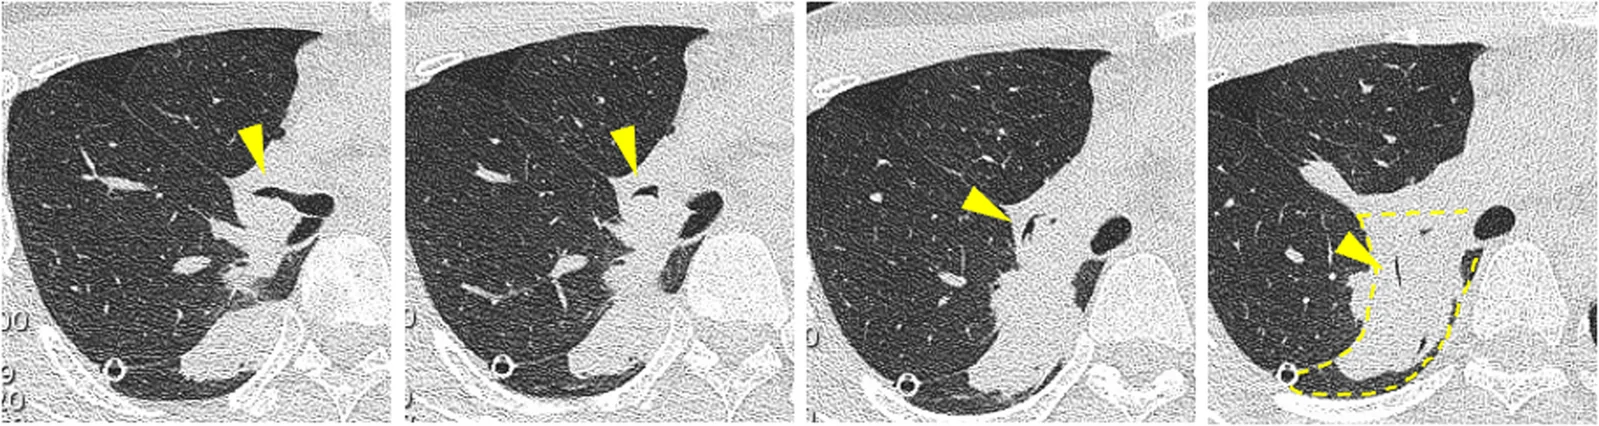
Chest computed tomography scan showed no obvious bullae. Atelectasis of the right middle lobe was observed, and the middle lobe bronchus extended posteriorly (arrow), indicating torsion of the middle lobe. The outline of the middle lobe is indicated by the dotted line
